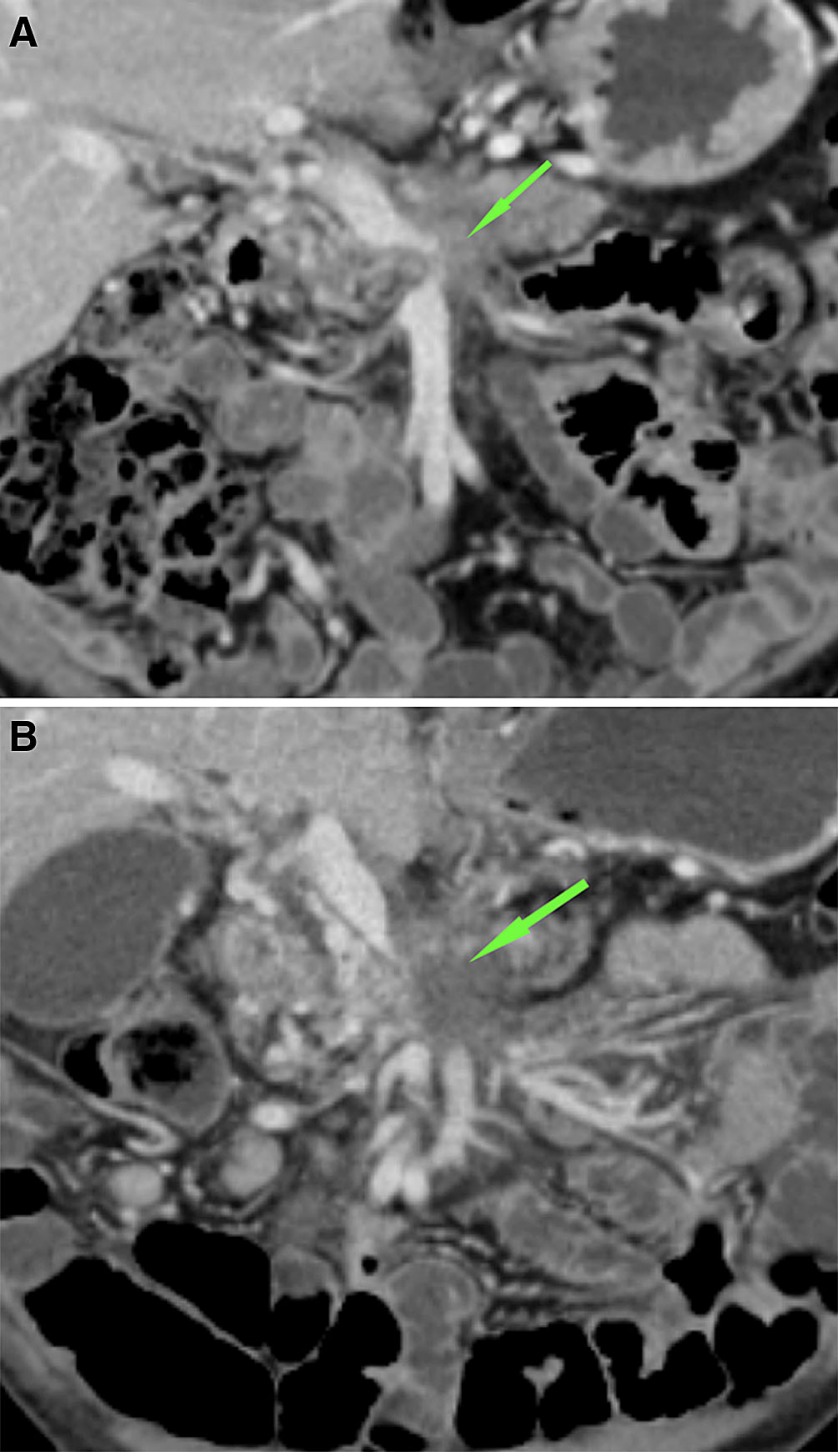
CT-images in the coronal plane, of the same patient as Fig. 6. A The superior mesenteric vein is already narrow on the pre-RFA imaging (arrow). B One week after RFA, the SMV is occluded for the part that is embedded in the ablation zone (arrow)
